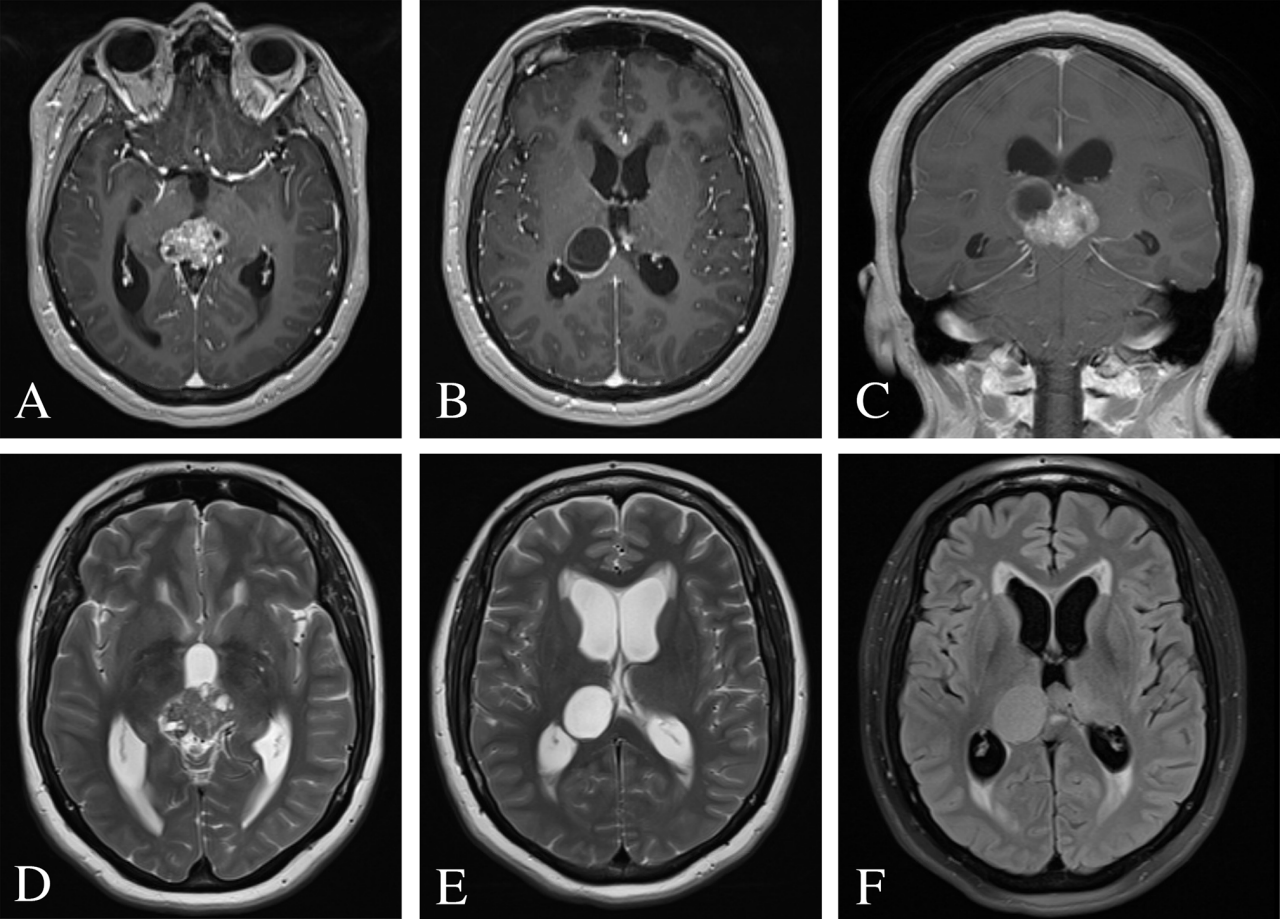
Pre-operative MRI of the brain. (A–C) Post-contrast T1-weighted images demonstrating a heterogeneously enhancing 3.9 × 2.6 × 3.1 cm mixed cystic and solid pineal mass and consequential obstructive hydrocephalus. (D, E) T2-weighted images revealed a hyperintense cystic lesion and hypointense solid lesion with mass effect and compression of the cerebral aqueduct resulting in supratentorial ventricular dilatation and periventricular white matter signal abnormality. (F) FLAIR image demonstrated periventricular transependymal flow of cerebrospinal fluid indicative of acute hydrocephalus.
